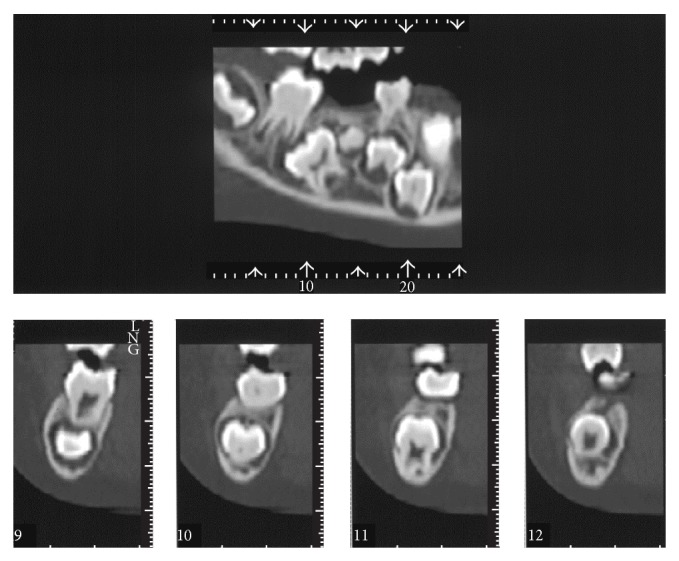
Sagittal reconstruction computed tomography: case  2. The root apex of the impacted primary molar is extremely close to the mandibular canal and mandibular plane.
